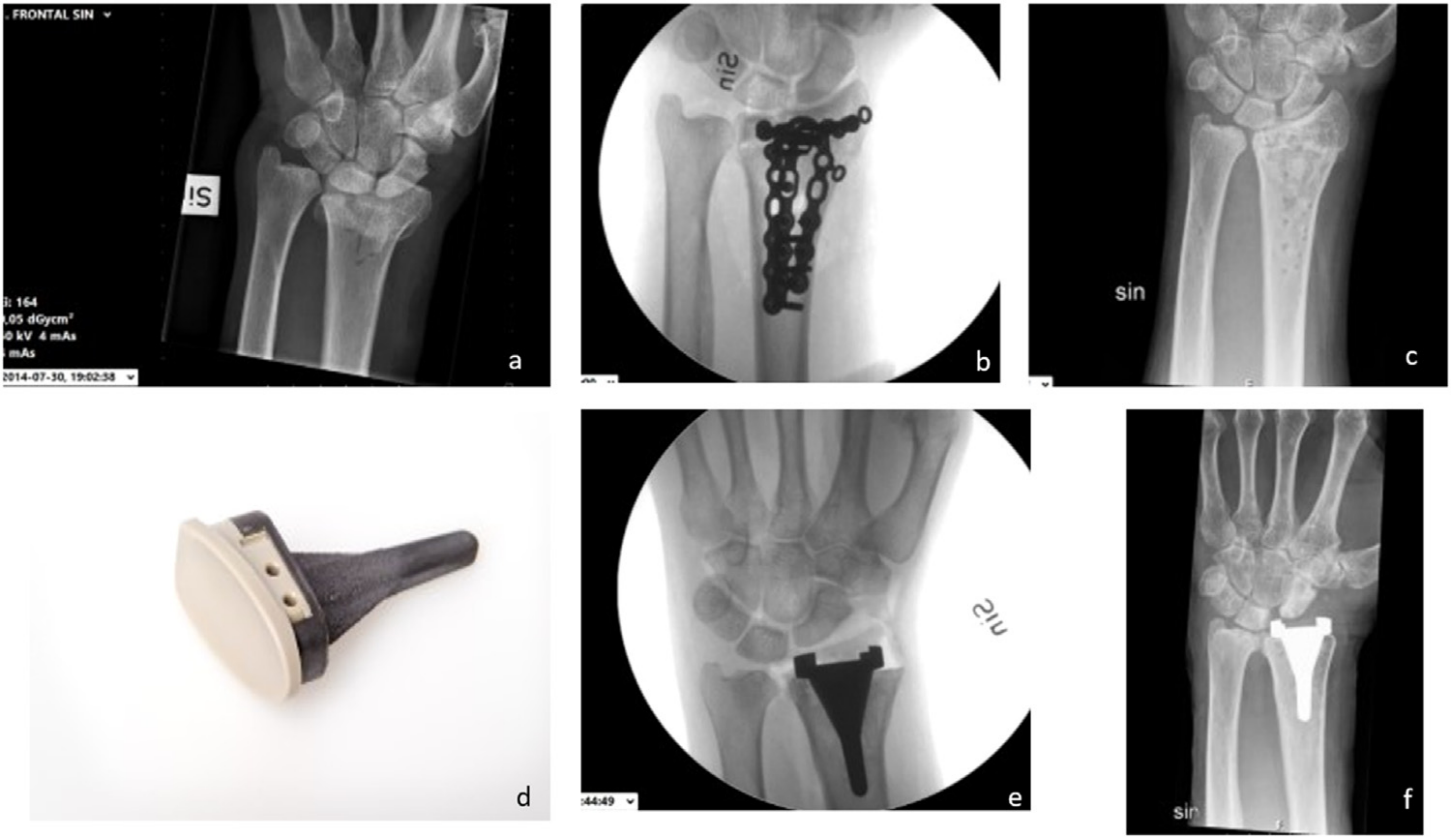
Initial radiographs: a) Distal radius fracture; b) fracture fixation with combined plating; c) after hardware removal; d) the new hemiarthroplasty design; e) perioperative radiographs after insertion of the hemiarthroplasty; f) radiographs one year postoperatively showing ulnar drift of the carpus and carpal impingement on the implant.
